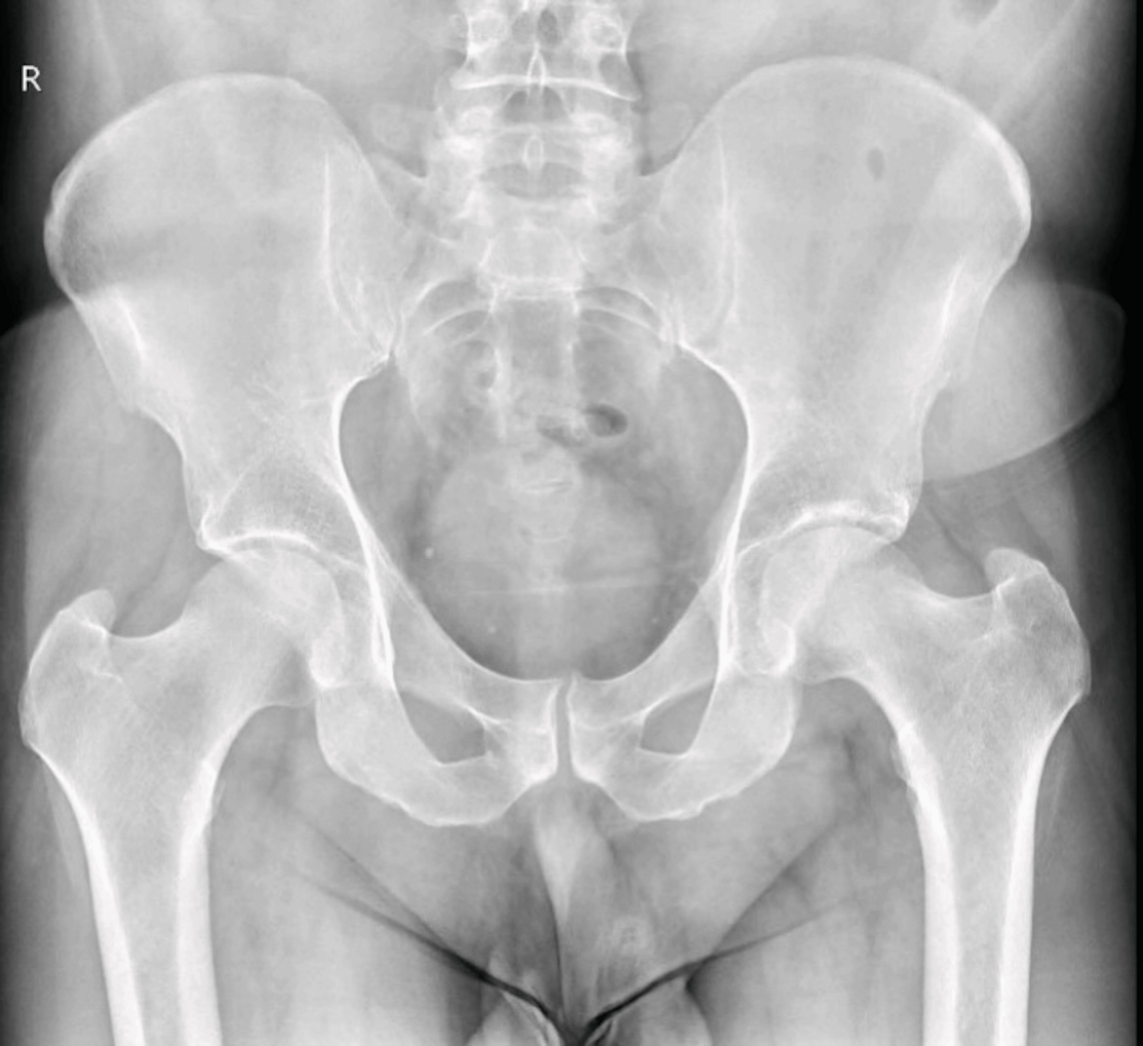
Anteroposterior radiograph of the pelvis showing no acute abnormalities at symptom onset
